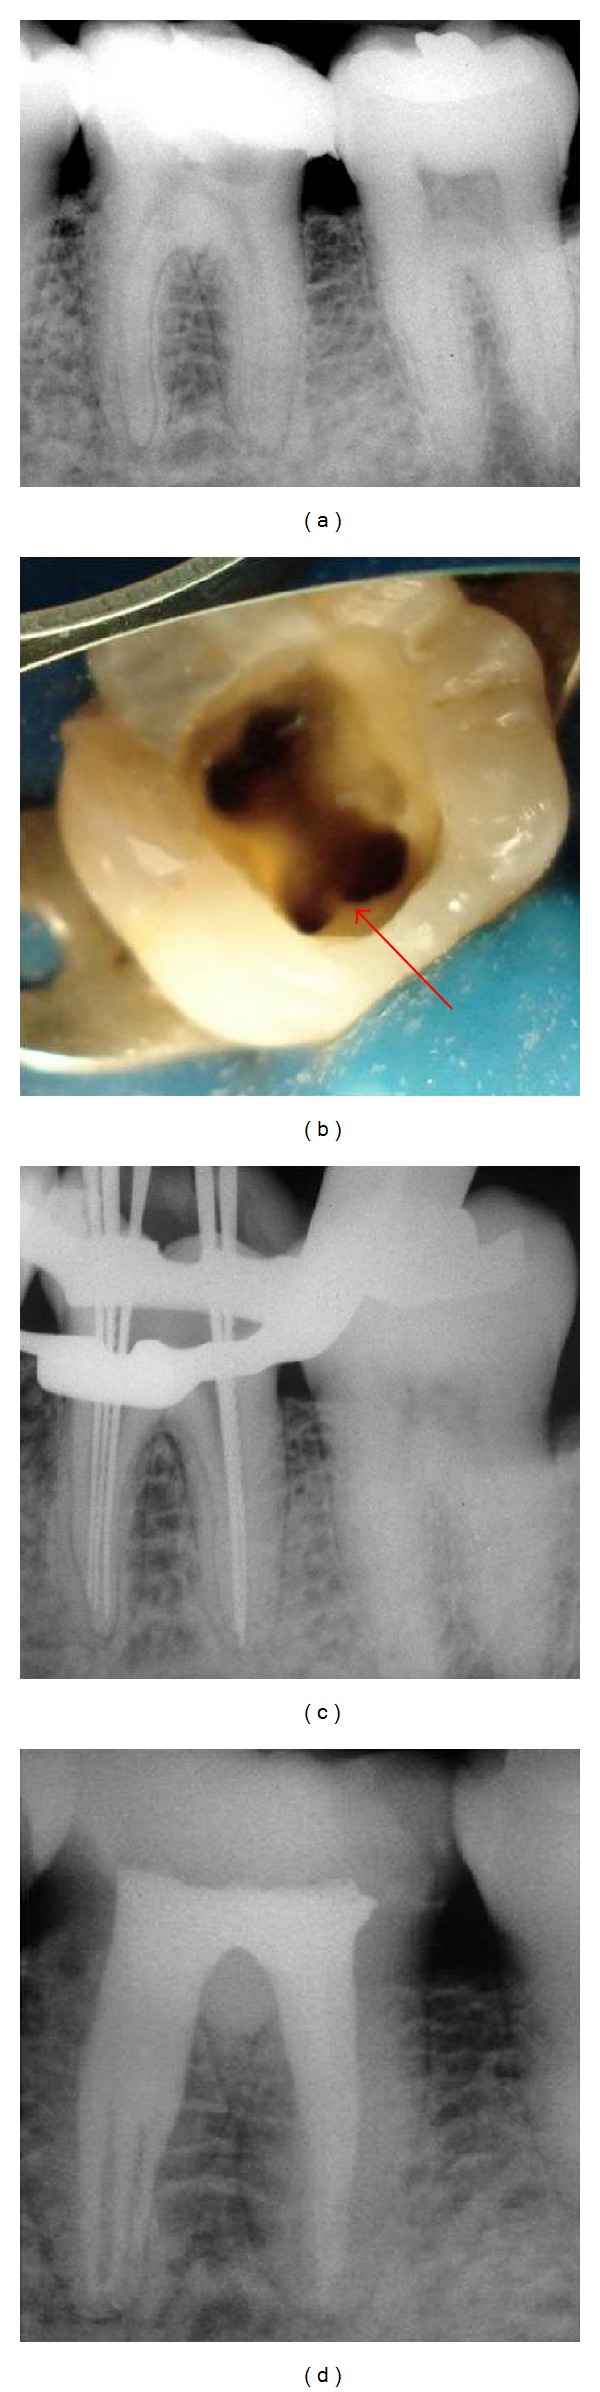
(a) Preoperative radiograph. (b) Clinical view of root canal entrances including a middle mesial canal (arrow). (c) Radiograph for working length determination showing three independent mesial canals. (d) Postoperative radiograph.
